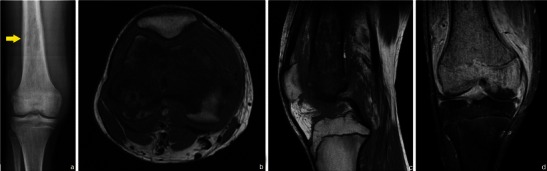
Acute osteomyelitis radiographic findings (a) can be aggressive and similar to those of Ewing’s sarcoma (permeating lesion with periosteal reaction, arrow in a). MRI however shows diffuse soft-tissue swelling, with no well-defined soft tissue mass (b axial T1WI; c sagittal T1WI; d contrast-enhanced T1WI)
